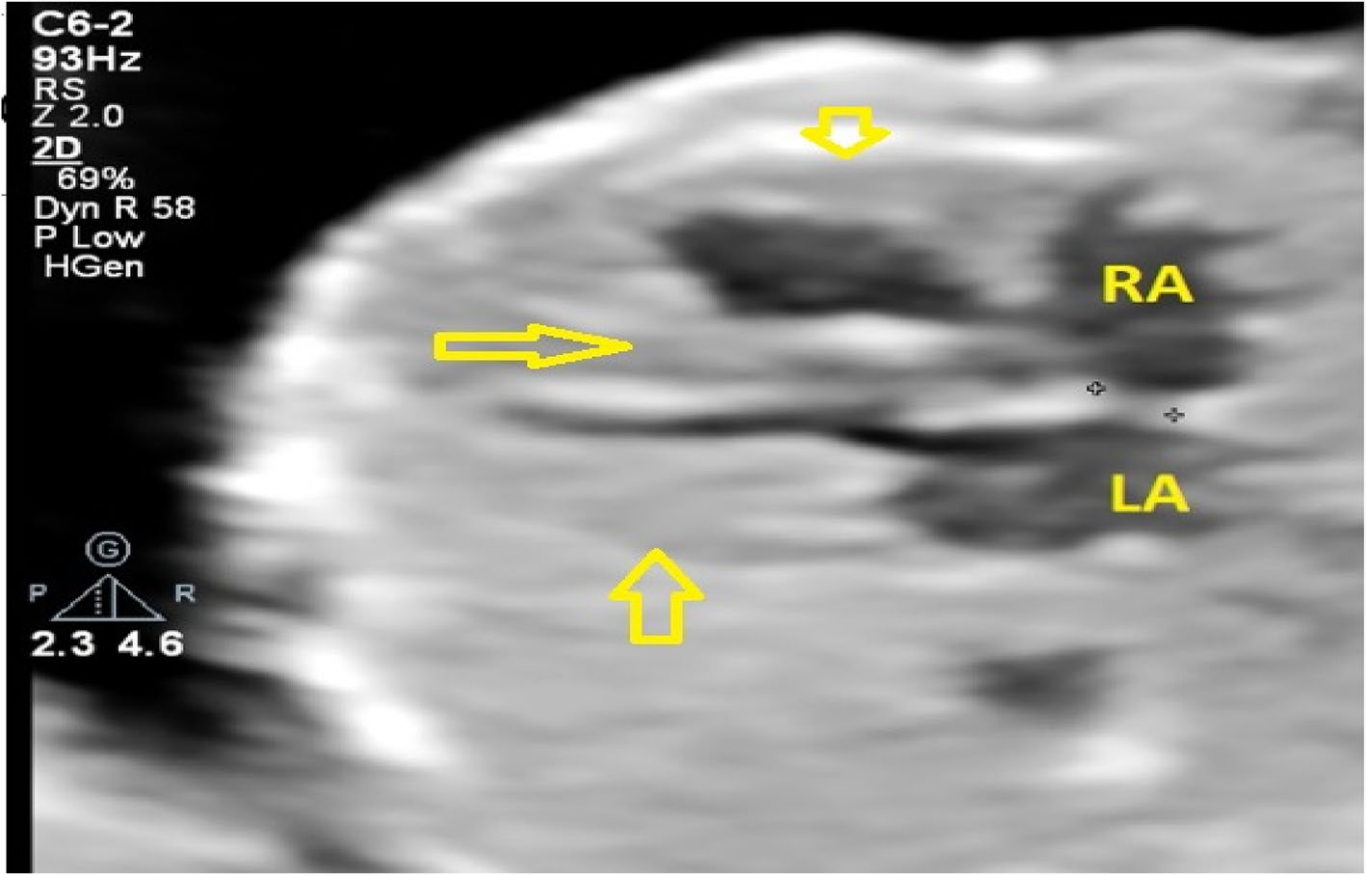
Subcostal four chamber view in fetal echocardiography shows biventricular hypertrophy (yellow arrow) and cardiomegaly
